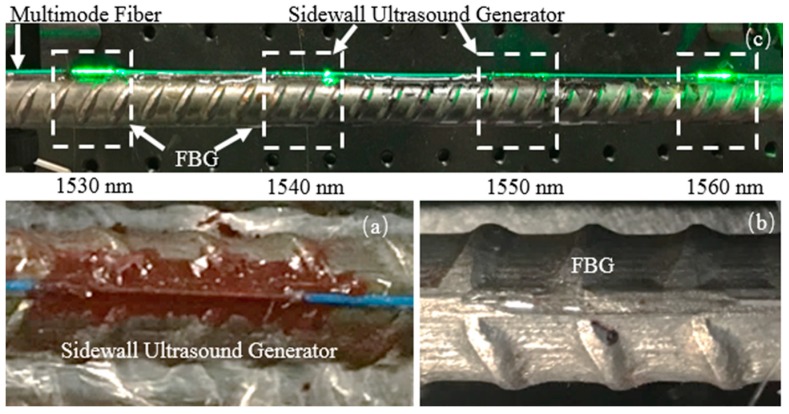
The fiber photoacoustic sensors. (a) The photo of the sidewall photoacoustic (PA) ultrasound generators attached on a rebar. (b) The photo of the fiber Bragg grating (FBG) detectors attached on a rebar. (c) The position of distributed photoacoustic sensors.
